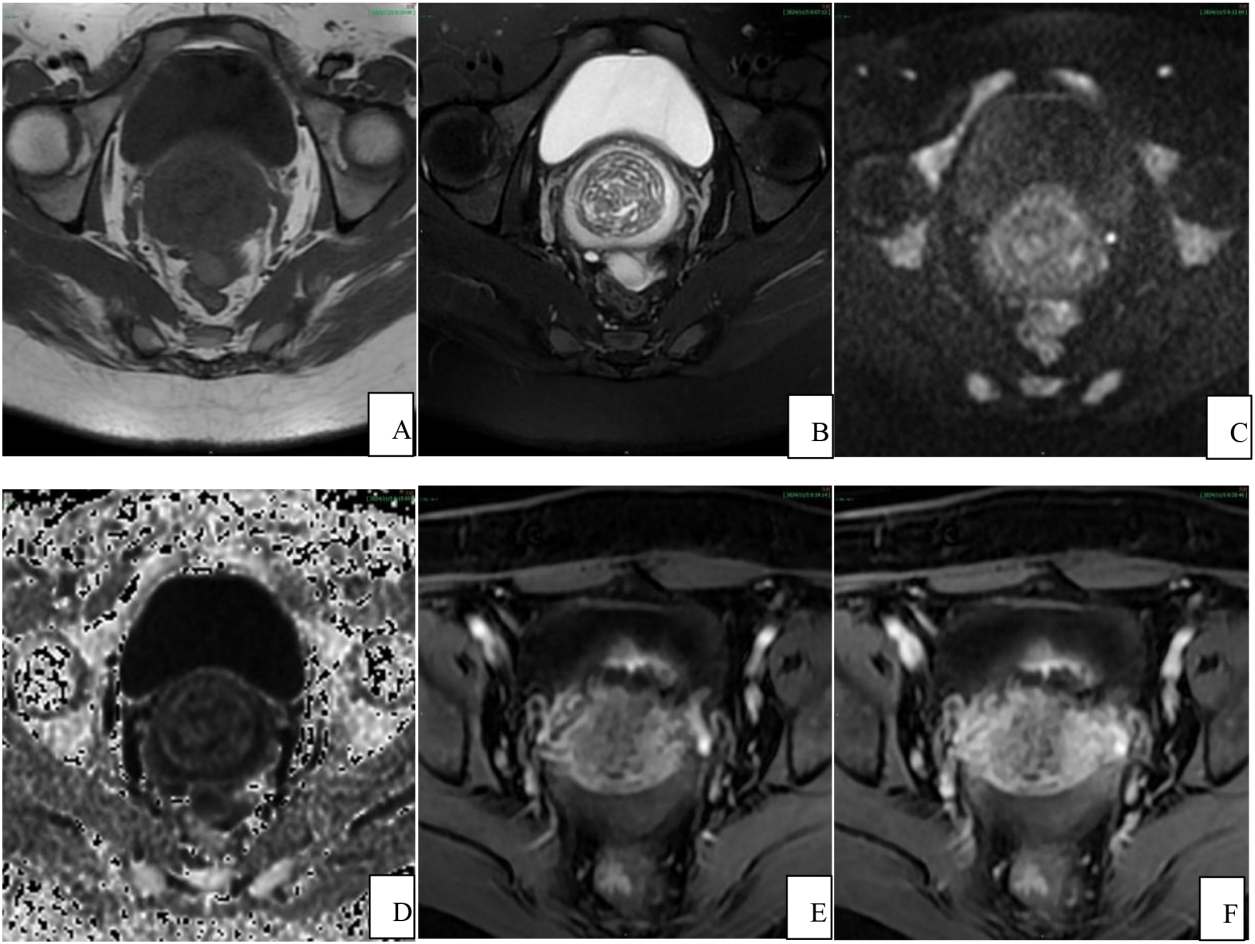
MRI axial scan results of the patient’s pelvic cavity. Diffuse multiple cystic lesions within the enlarged cervical wall, with unclear boundaries and no restricted diffusion. The cyst wall is enhanced on contrast-enhanced scans, while the cyst interior remains unenhanced. The lesions have invaded the subserous layer. (A) T1WI; (B) T2WI; (C) DWI; (D) ADC; (E) arterial phase after contrast enhancement; (F) delayed phase after contrast enhancement.
